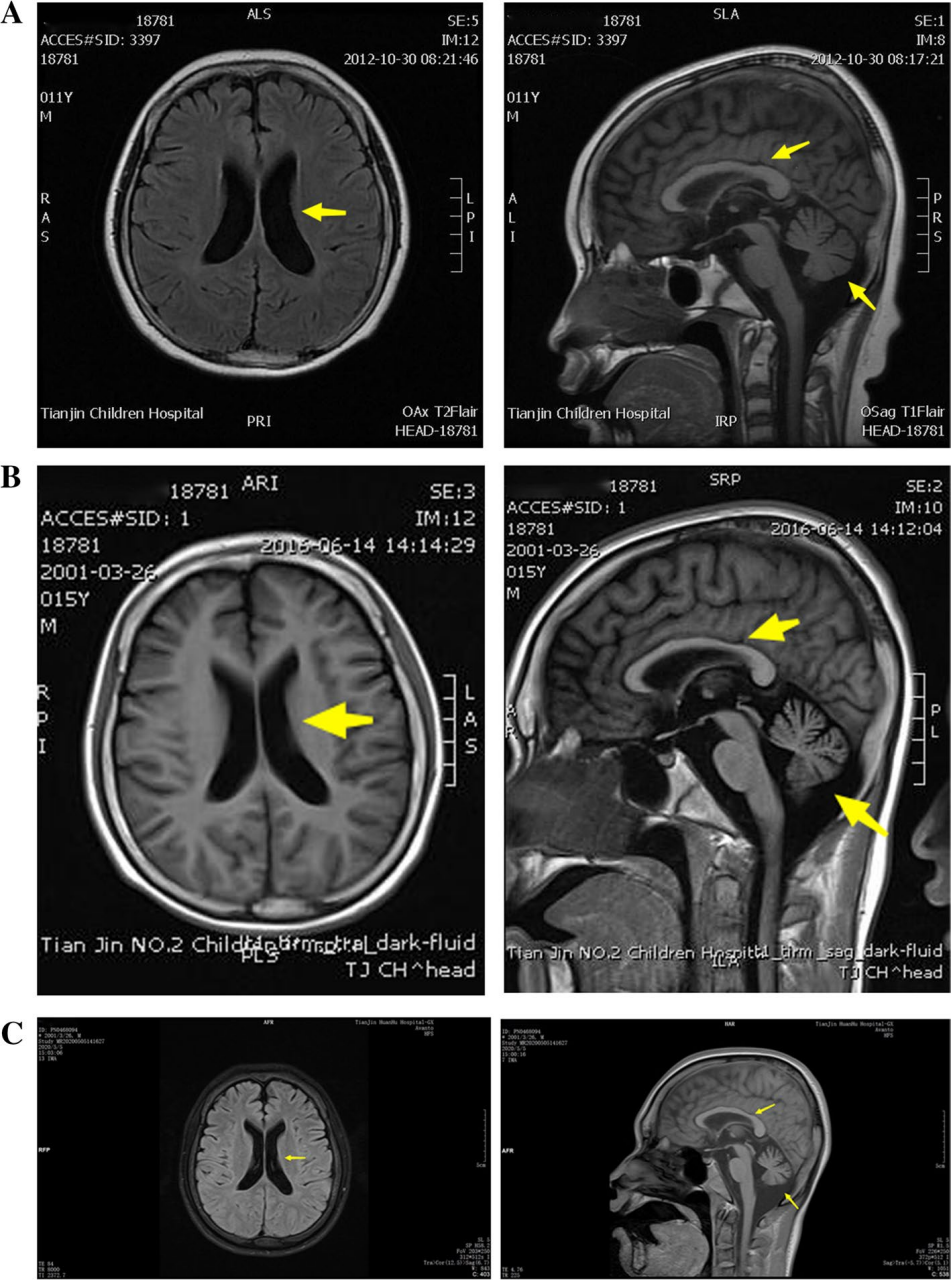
Brain MRI findings. the brain MRI showed mild enlargement of lateral ventricle, corpus callosum was normal, and partial widening of the cerebellar sulci in 2012 (A); enlargement of lateral ventricle, partial thinning of corpus callosum, significant enlargement of the cerebellar sulci in 2016 (B); Cerebellar atrophy and thinning of corpus callosum were more serious than before in 2020 (C)
